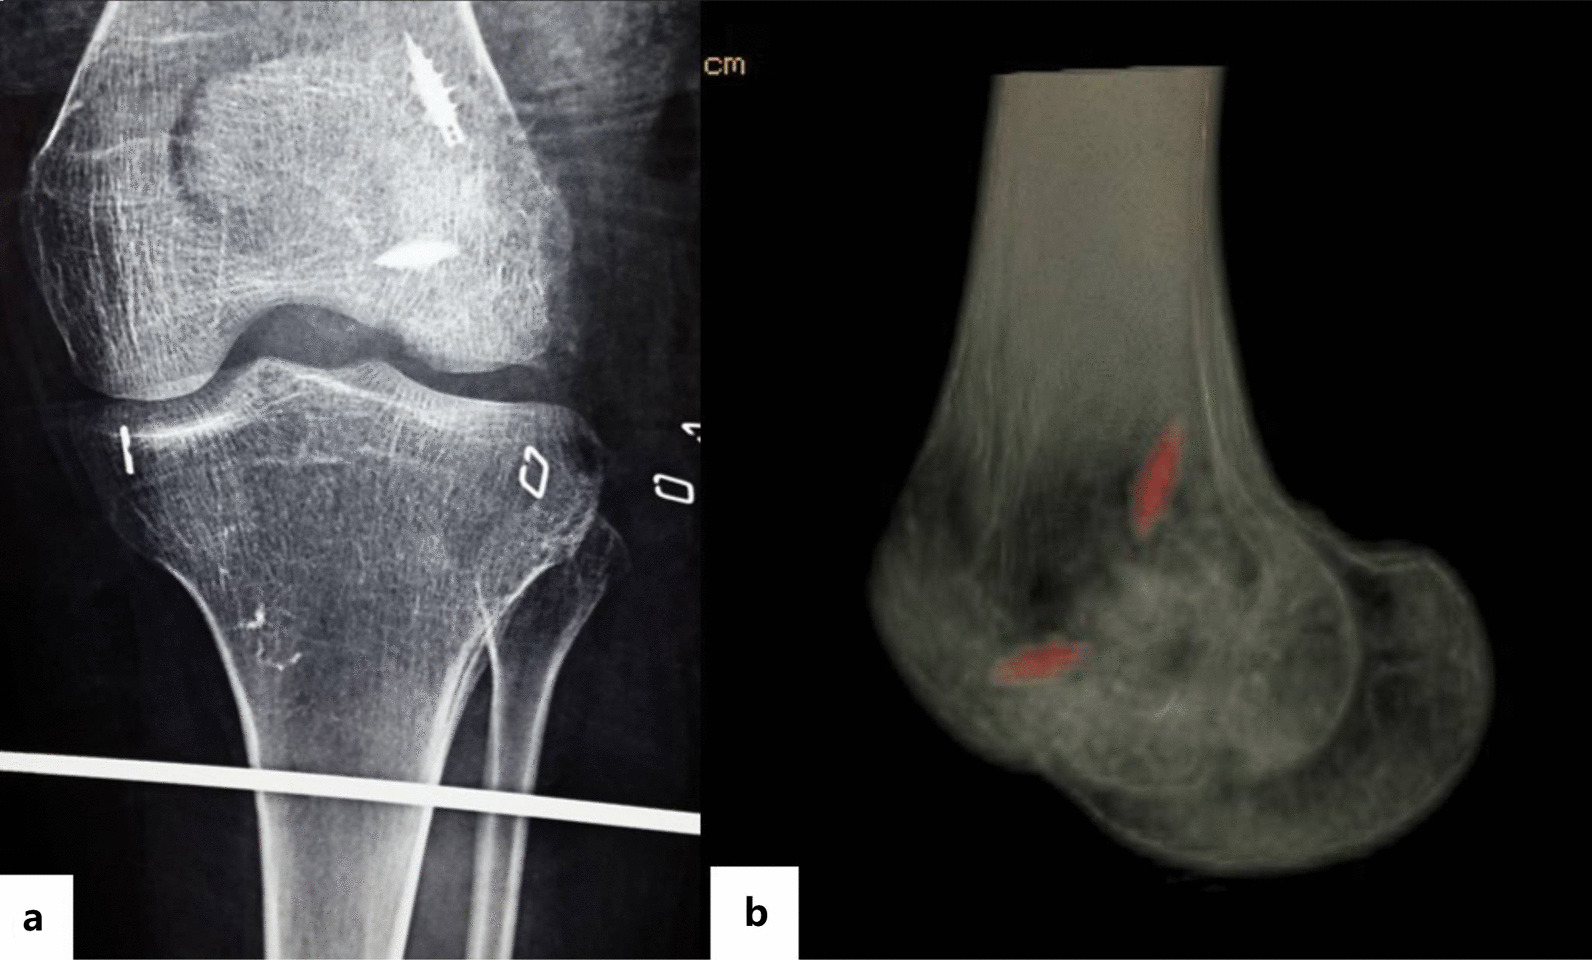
Postoperative tibial tuberosity traction was performed (a), and CT showed good positional alignment of fracture (b)
